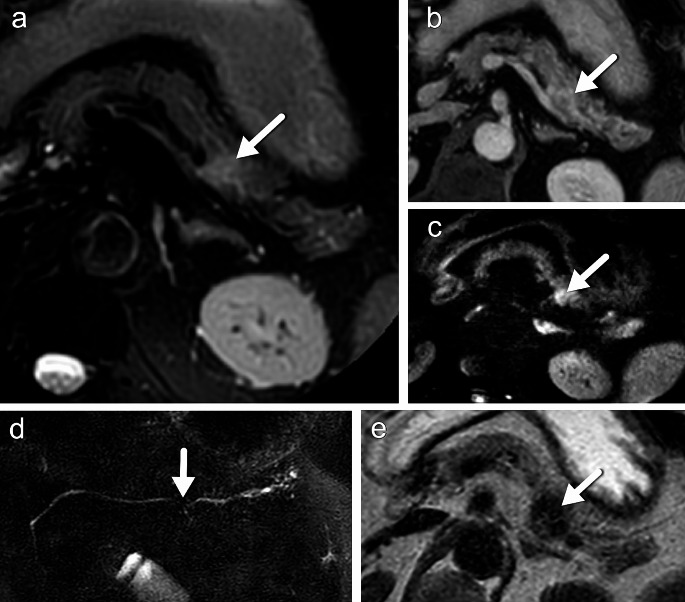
12 mm PDAC in the pancreatic tail on fat suppressed T2 (A), late enhancement on T1 after contrast (B), DWI (b800) (C), MRCP (D), T1 turbo field echo (E)
